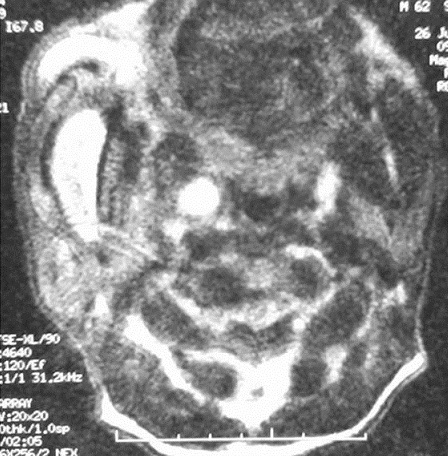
Cervical MRI axial FAT SAT T2 section showed abscess 40 x 20 mm of the right parotid gland (black arrow)
